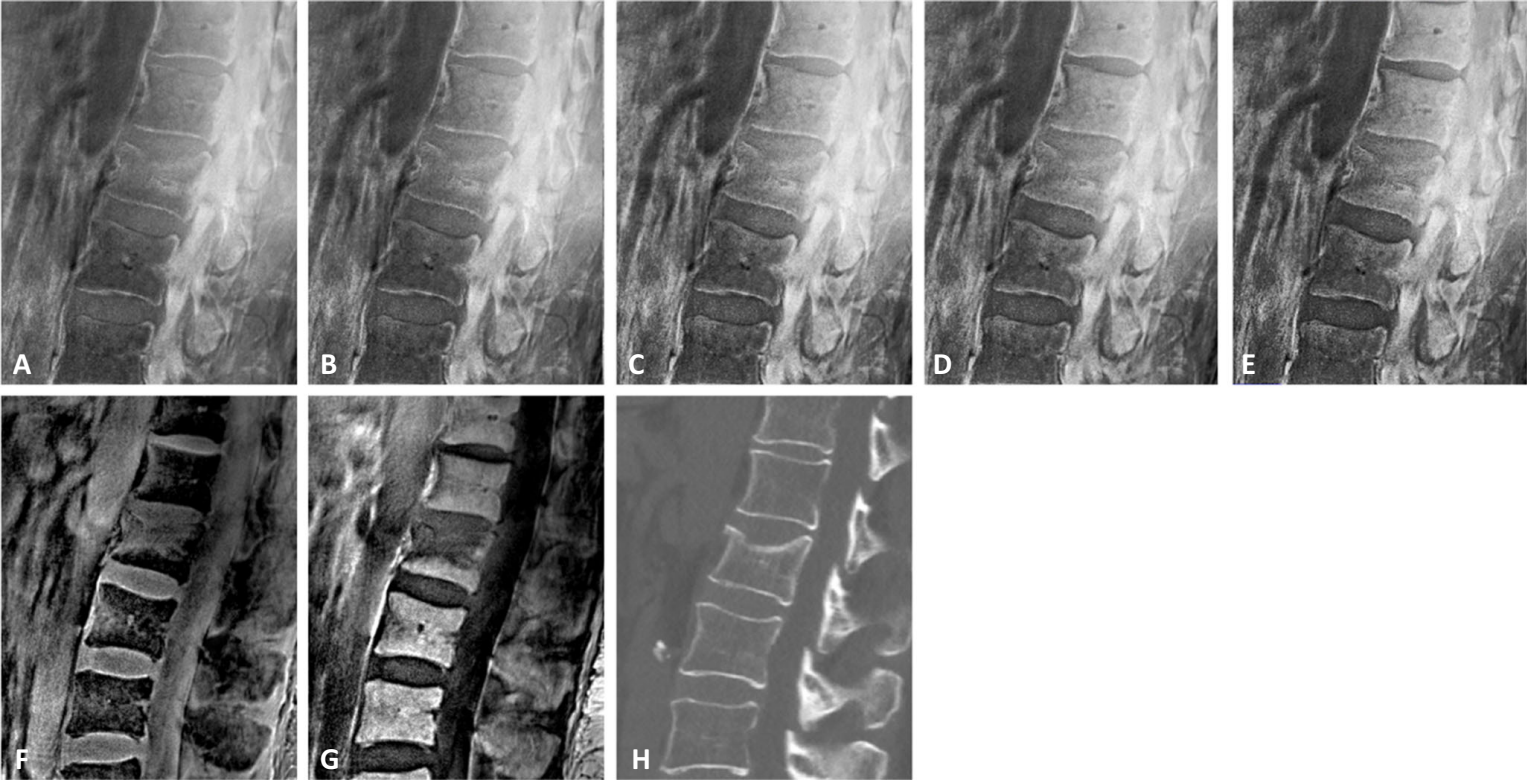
UTE-derived SW-like images with CT-like contrast and corresponding water- and fat-separated images (A–G) showing an acute compression fracture in L2. For postprocessing of SW-like images, the input UTE phase was corrected and unwanted phase terms were removed. Afterwards phase masks were applied to the UTE magnitude image to increase the contrast of areas with negative phase. Note the increasing contrast of the vertebrae with the increasing number of phase masks applied (A
